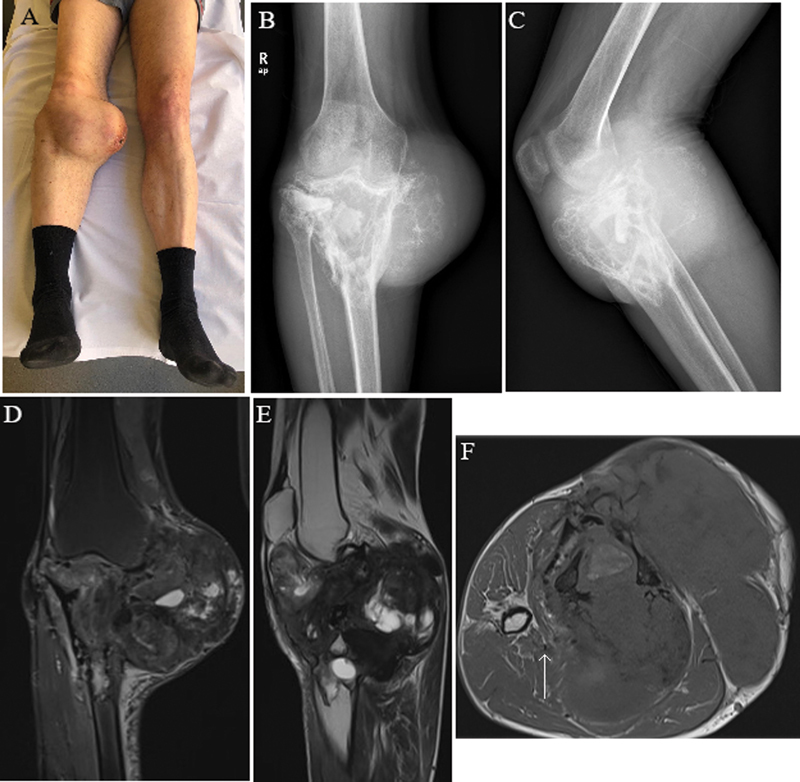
Preoperative images of the patient. A) The clinical photograph shows an evident knee deformity, with a hard palpable medial mass measuring 10cm. B and C) Radiographic evaluation shows a massive lytic tumor in the proximal tibia. The lesion presented an aggressive growth with bone destruction, trabeculation and extension into the fibula and the surrounding soft tissue. D, E and F) MRI images demonstrated a 13 × 15 × 13 cm mass (AP × ML × CC) with significant soft tissue involvement and displacement of neurovascular structures (white arrow). Heterogenous contrast enhancement can be observed.
